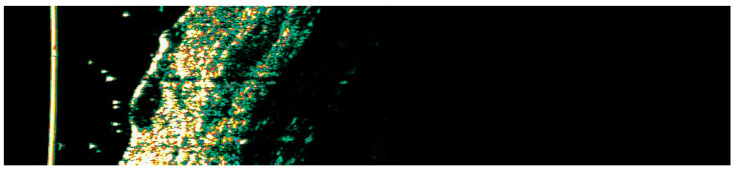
Ultrasonographic image of BCC on the chest.
